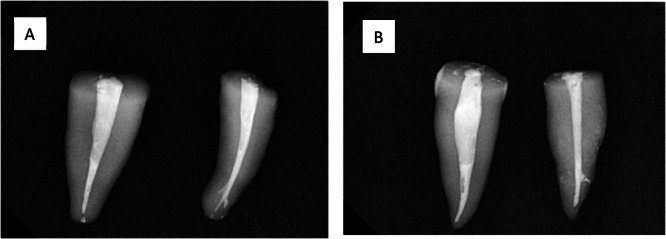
Radiographs of the obturated teeth: (A) samples processed at 200°C and (B) samples processed at 120°C.
